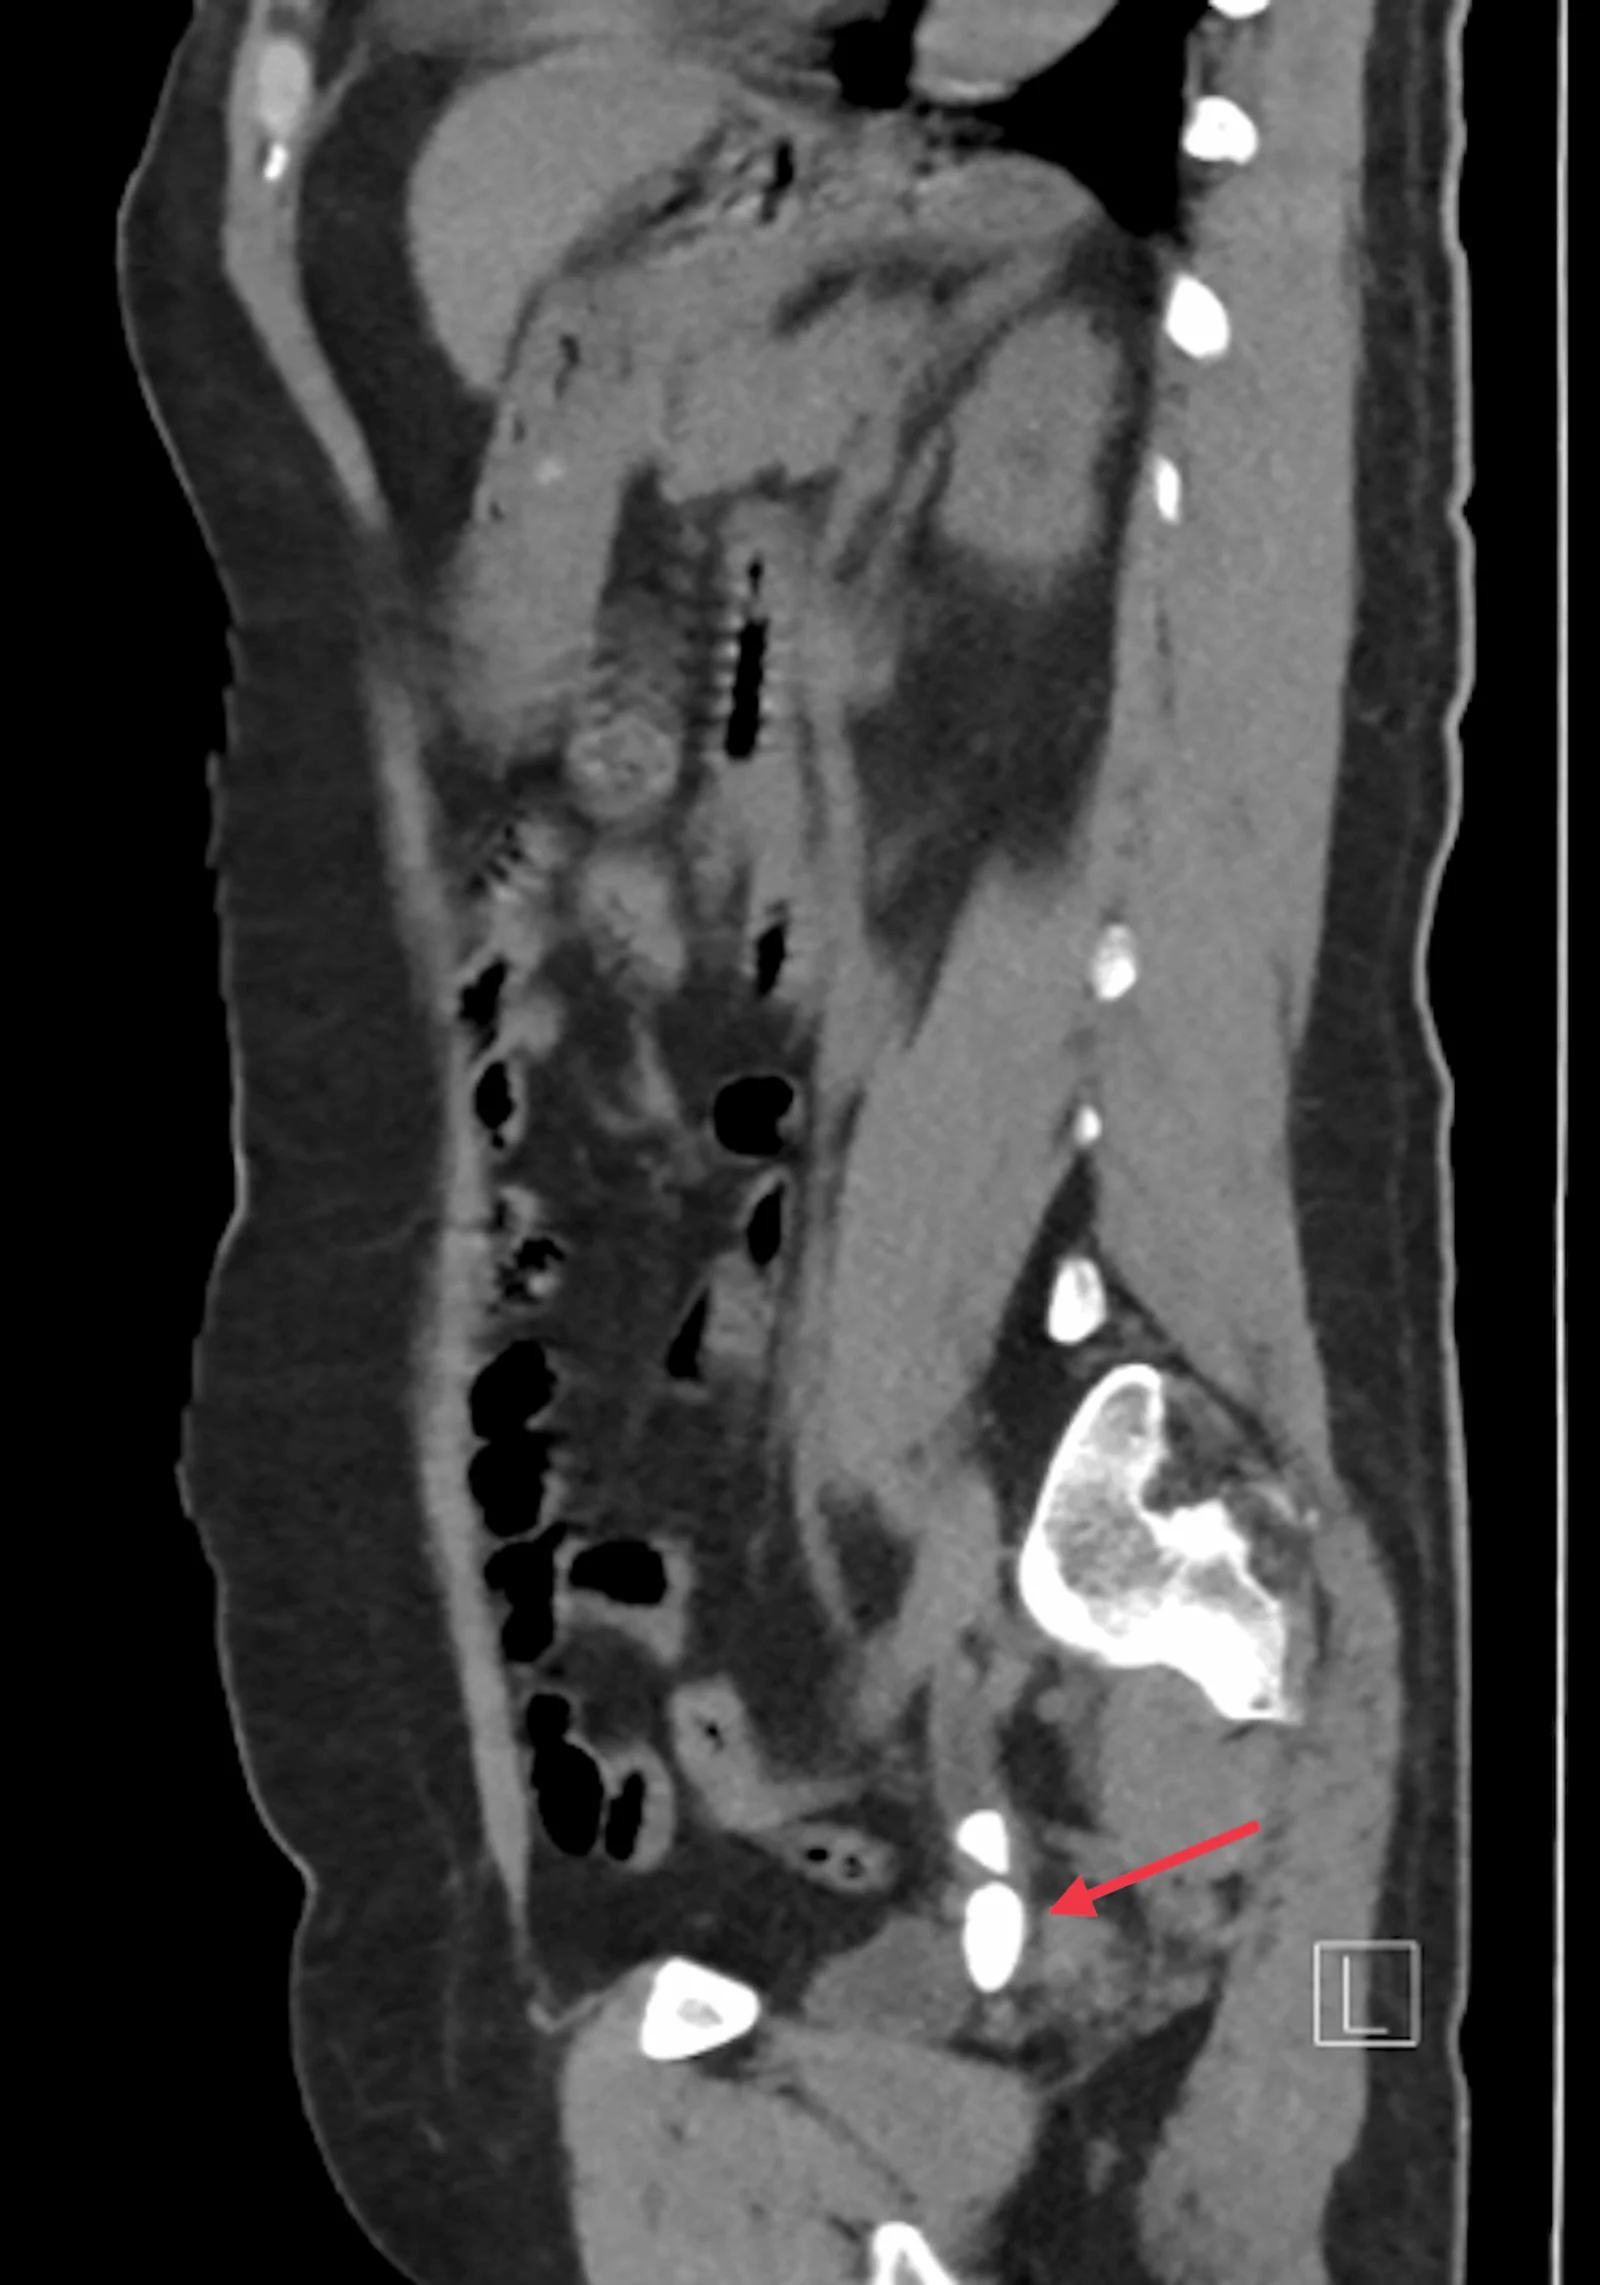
Sagittal non-contrast CT image showing left distal ureteral stones. The red arrow points to the stone.
